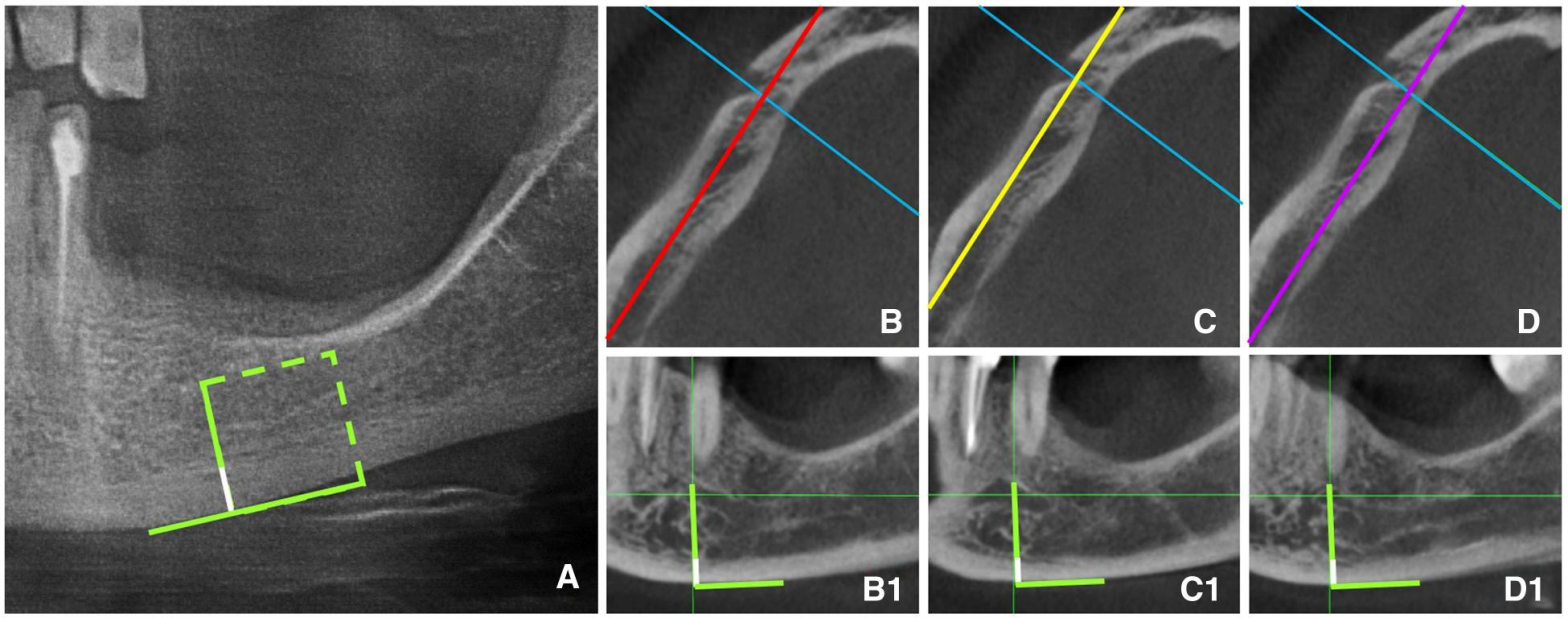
Measurement of MCT in PR and three sagittal CBCT slices. (A) PR image of the hemi-mandible showing the perpendicular line drawn to the tangent line to the inferior border. (B, B1) Measurement of MCT in the sagittal CBCT slice passing through the midpoint of the buccal and lingual cortical plates of the mandible, and the sagittal slices. (C, C1) 2 mm to the buccal, and (D, D1) 2 mm to the lingual to the middle slice. MCT was measured in the inferior part of the perpendicular line (white lines) for PR and each CBCT slice.
